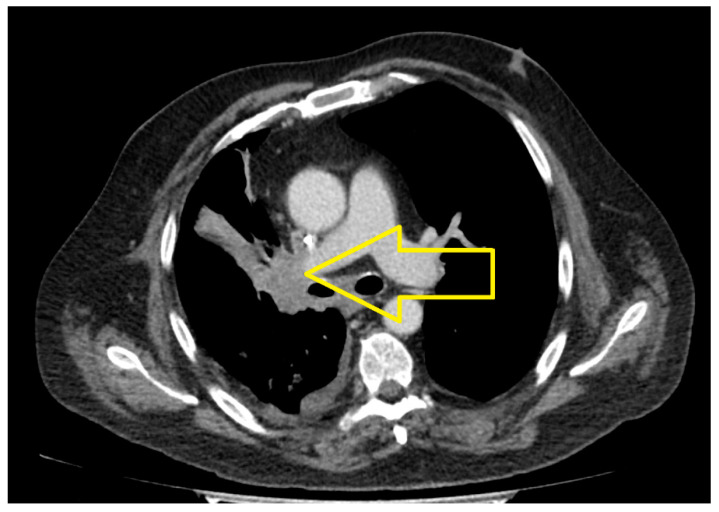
Chest CT (June 2024): Lung metastases located apically and right supra-hilar, with maximum axial diameters of 85/22 mm and 42/20 mm, respectively, in progression compared to June 2023.
